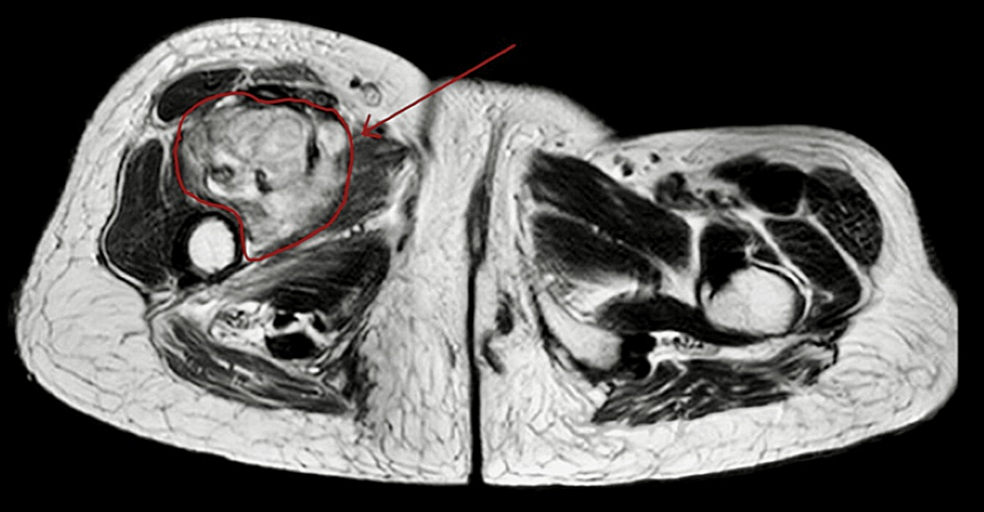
T2-weighted image showing a heterogeneously hyperintense lesion in the intramuscular plane of the right thigh (red arrow).
